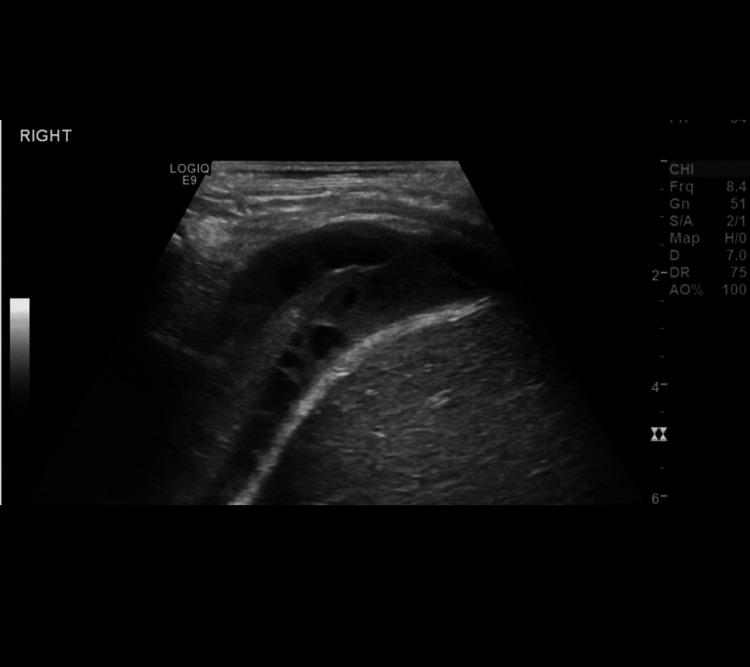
Ultrasound examination in pleural effusion with septations
